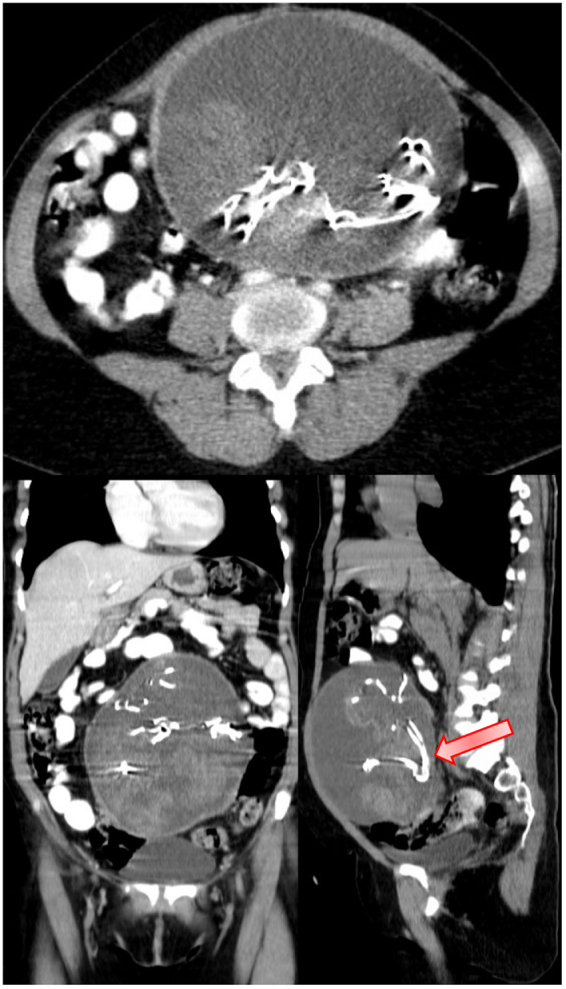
Contrast enhanced computed tomography (CT) of the abdomen and pelvis in a 56-year-old postmenopausal woman with a history of total abdominal hysterectomy.
